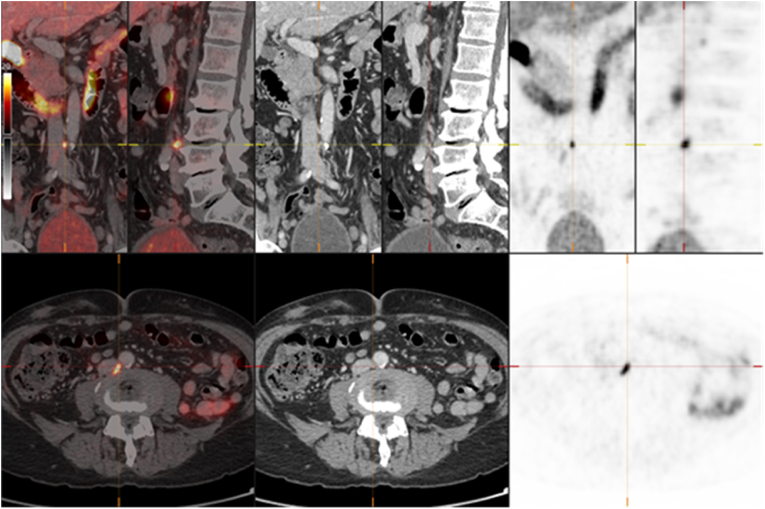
300MBq ^18^F-PSMA-1007 i. v., imaging after 120 minutes with contrast enhanced CT, Colour scale Hot Metal 0–20, PET/CT, CT and PET images in coronal, sagittal and axial plane. Metastatic lymph node marked with crosshair. (For interpretation of the references to colour in this figure legend, the reader is referred to the Web version of this article.)
